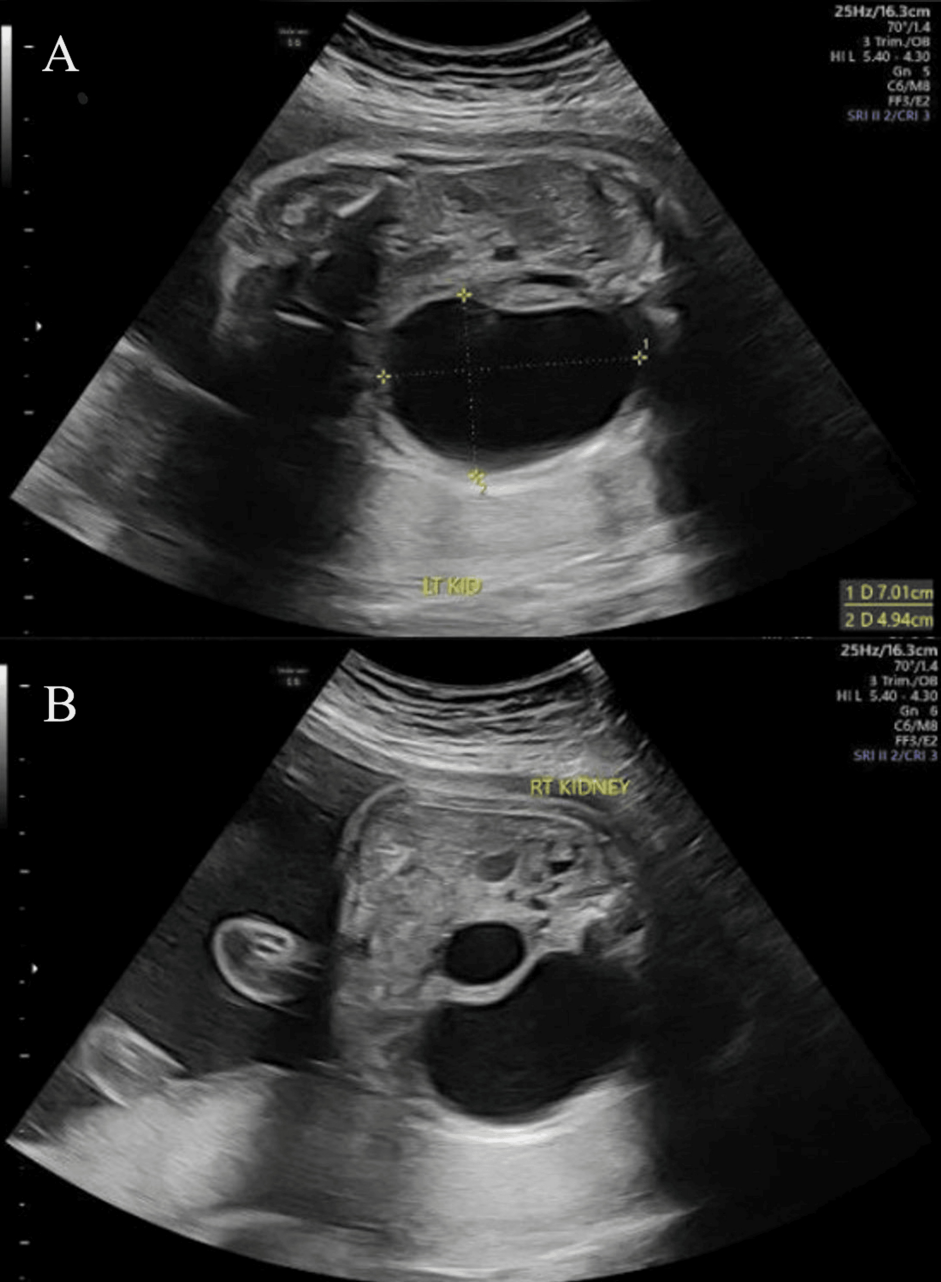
Prenatal abdominal ultrasound demonstrating the fetal kidneys in transverse view. (A) The left kidney (LT KID) appears enlarged with multiple non-communicating cystic spaces of varying sizes and loss of normal corticomedullary differentiation, with the largest measuring 7.01 × 4.94 cm. This is consistent with the diagnosis of MCDK. (B) The right kidney (RT KIDNEY) is shown in comparison with no abnormalities. MCDK: multicystic dysplastic kidney
